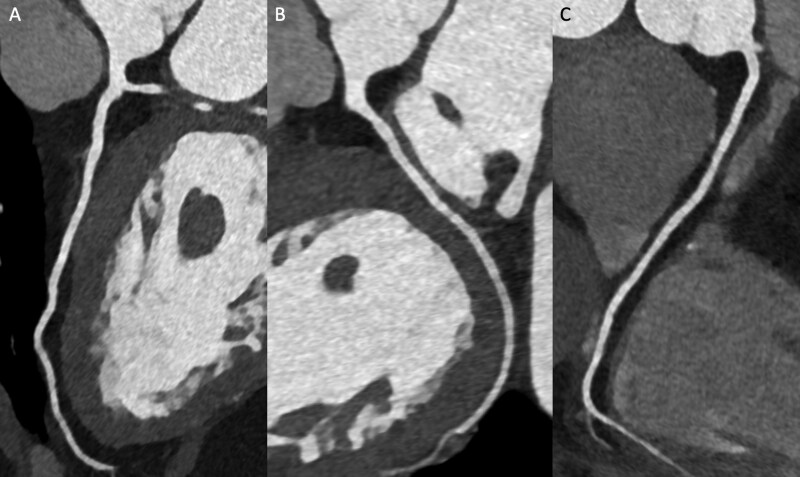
Coronary CTA with the FLASH protocol. Single best-diastolic-phase acquisition with high quality images of the LAD (A), LCX (B) and RCA (C). This is the preferred scanning option for a patient in sinus rhythm and heart rate <65 bpm without coronary calcification on the planning scan.
